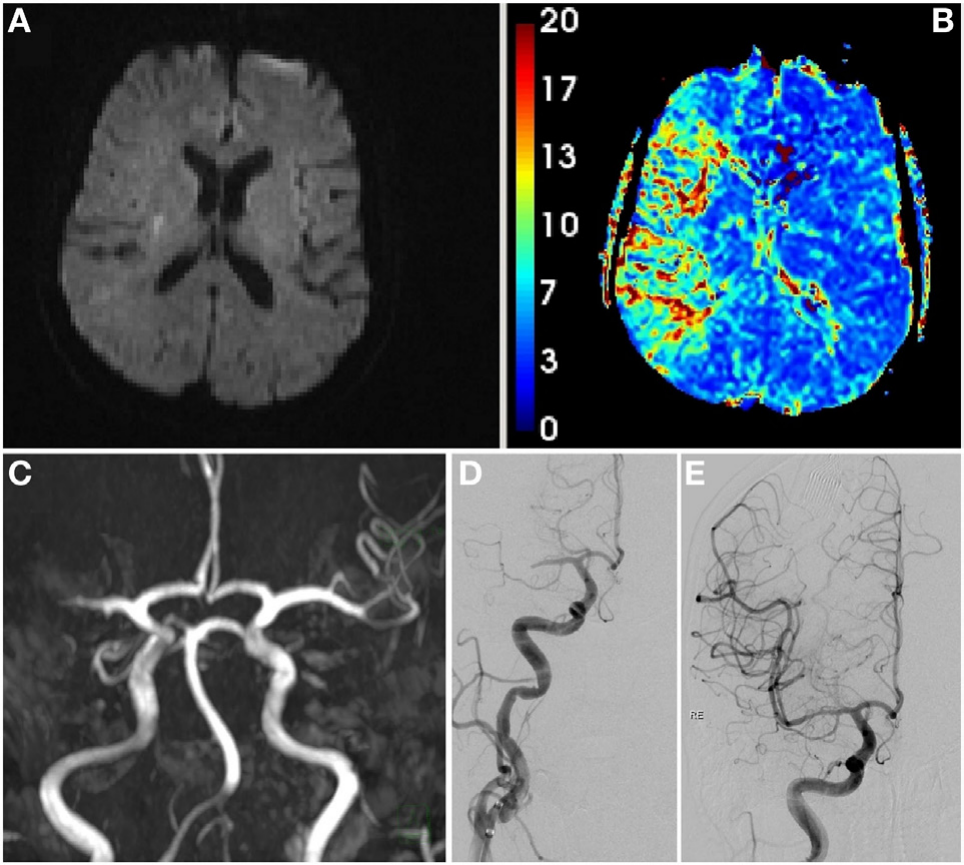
Initial MRI scan at second admission showing only a small DWI lesion corresponding to the preceding stroke (A), hypoperfusion of large parts of the middle cerebral artery (MCA) territory [(B), mean transit time], and right proximal MCA occlusion [(C), time-of-flight angiography]. Digital subtraction angiography pre- (D) and post-thrombectomy (E).
